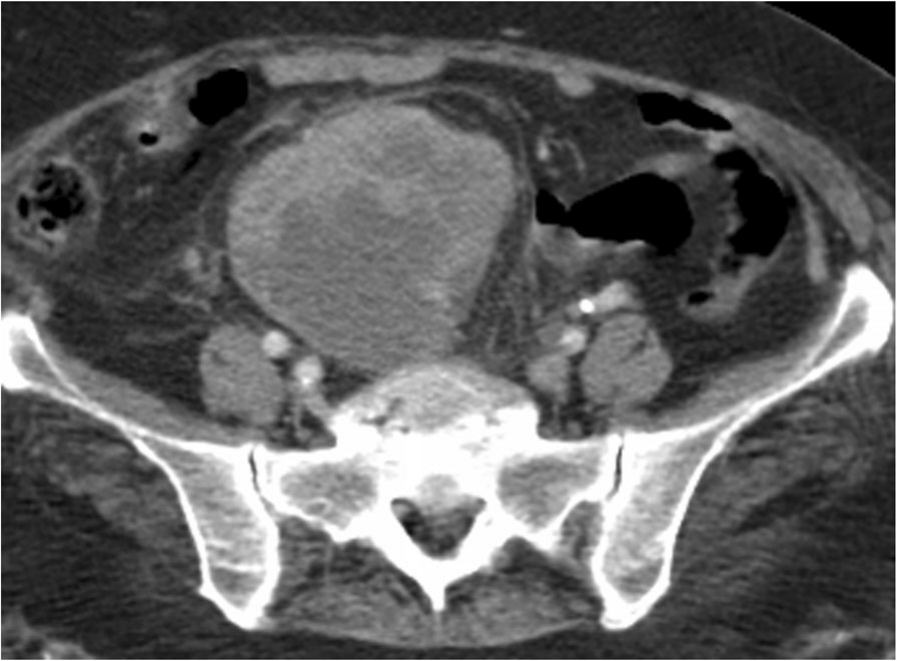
Contrast-enhanced computed tomography. There are hypodense areas in the pelvic kidney parenchyma with irregular limited contrast enhancement. The renal parenchyma shows heterogeneous and minimal contrast enhancement. There is no obvious mass lesion. The findings were consistent with chronic pyelonephritis
